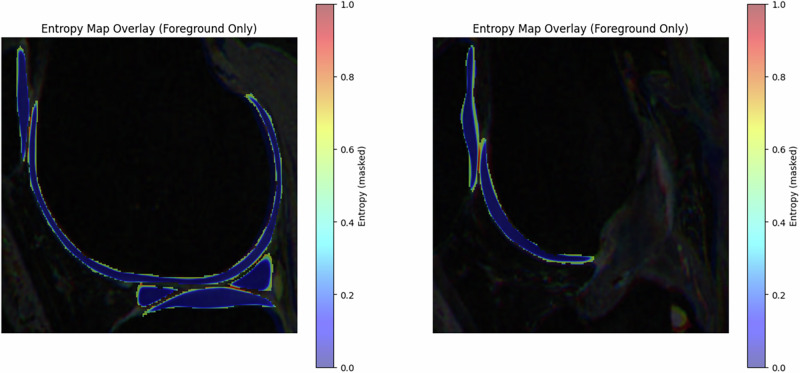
Entropy-based uncertainty maps for AI explainability (two representative examples). The map is computed from the fused softmax outputs of four independently trained models and overlaid on the input MRI slice. Warmer colors indicate high uncertainty, while cooler colors represent confident predictions. Background pixels are masked to focus on uncertainty in foreground anatomical structures.
